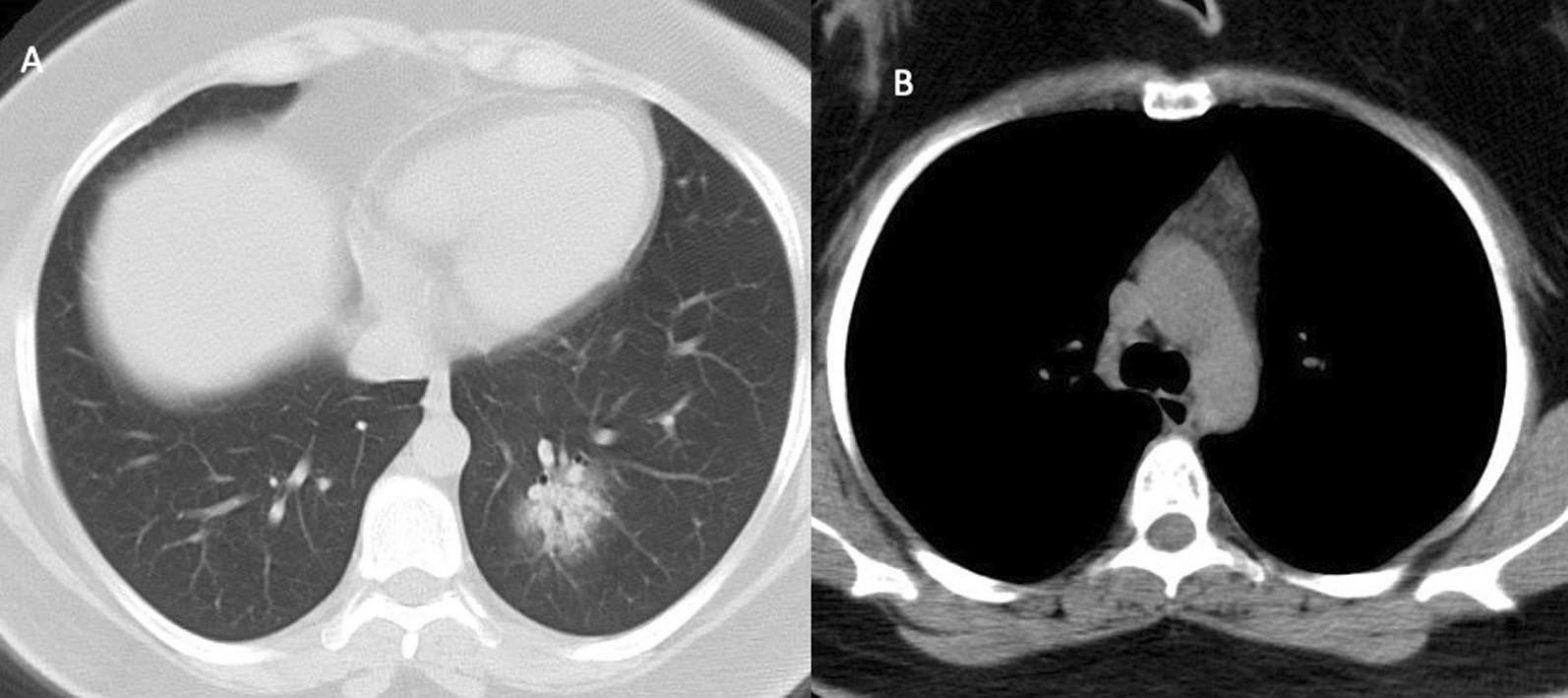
26-year-old female COVID-19 (+) patient with CT-SS 1 (A) and nodular-type thymus (B)
